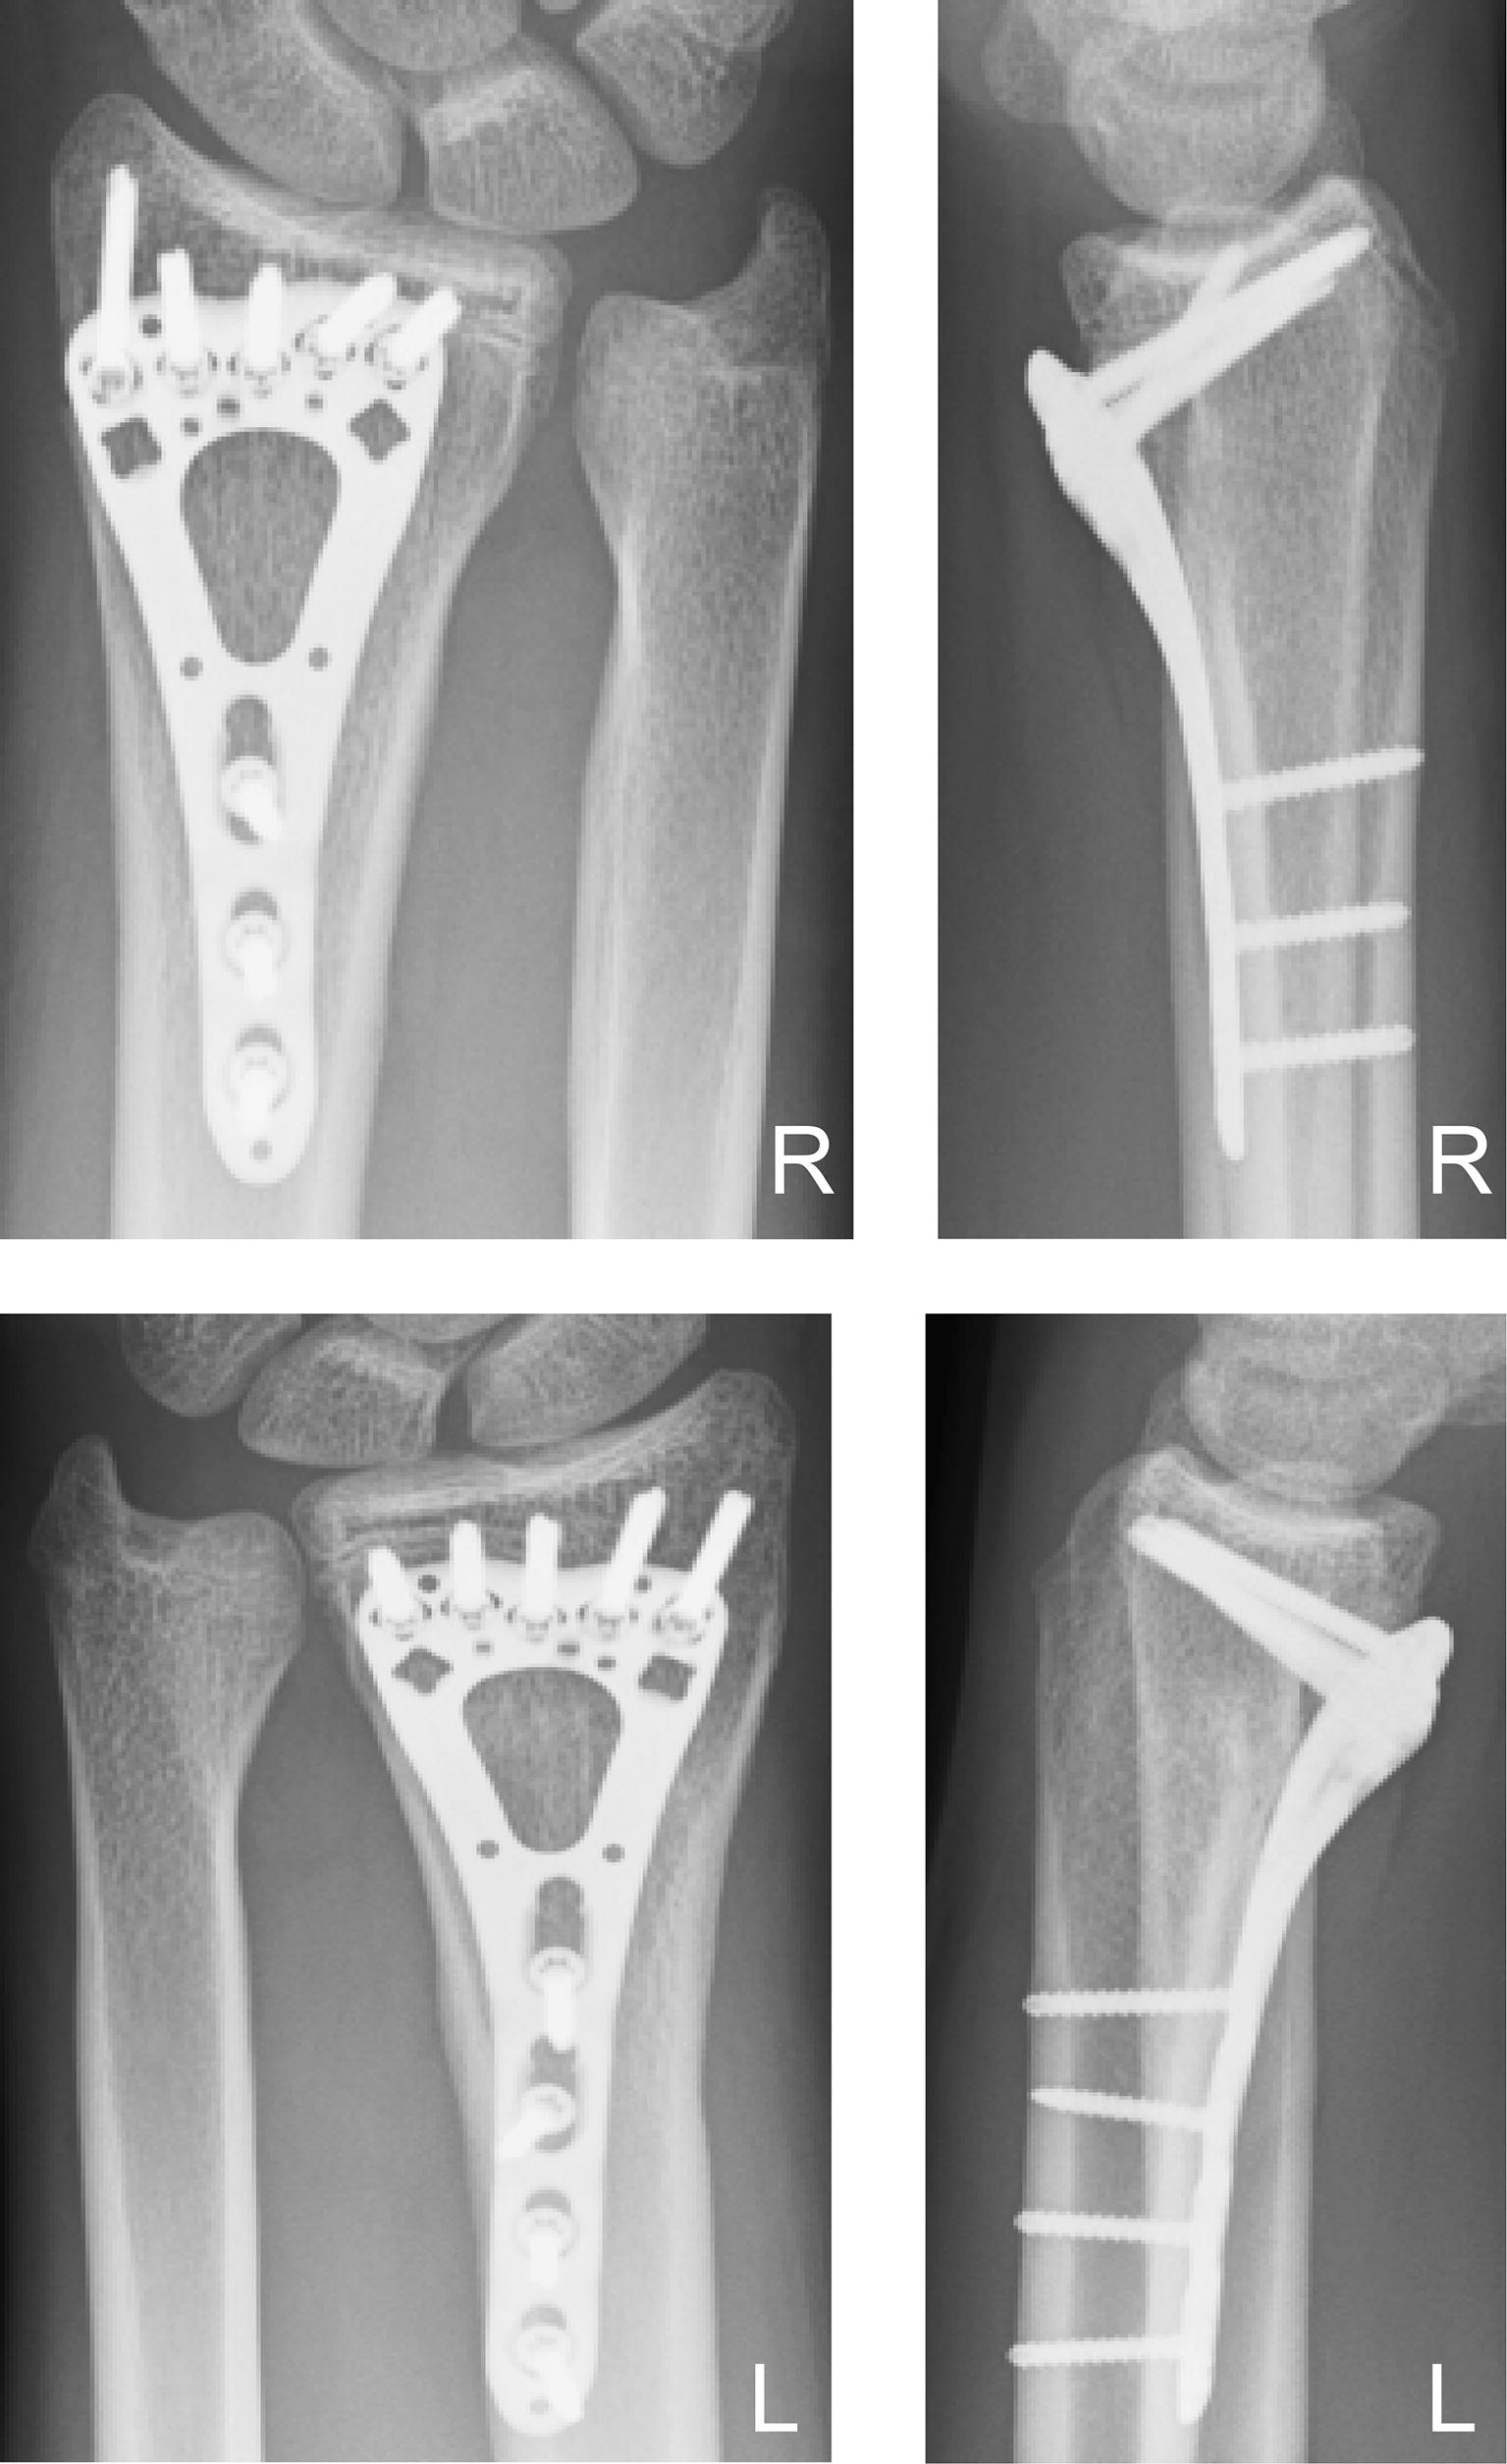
Follow-up imaging case 2. Radiographs showing healed fractures 3 months postoperatively
